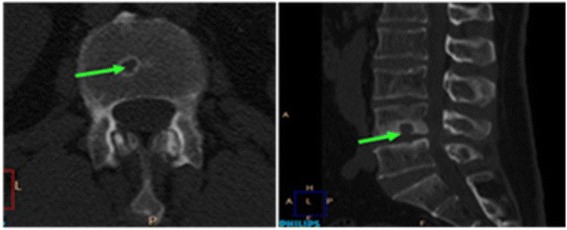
Schmorl’s node as seen on CT scan. Axial (left) and sagittal (right) sections
